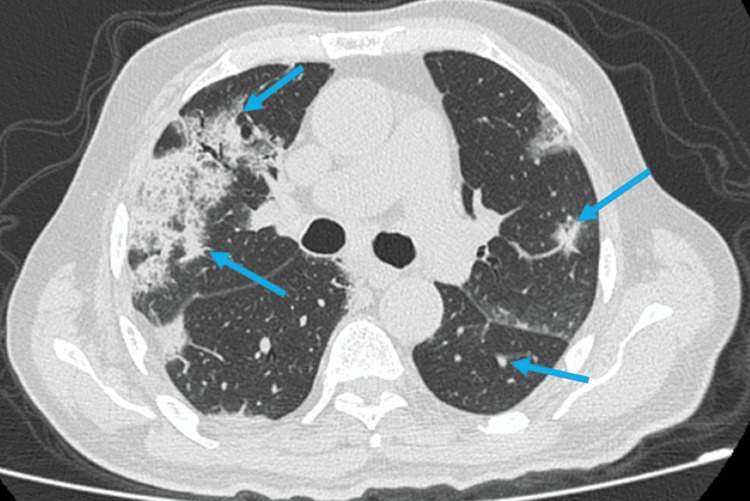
CT chest showing isolated infiltrates and consolidation in both lungs.
